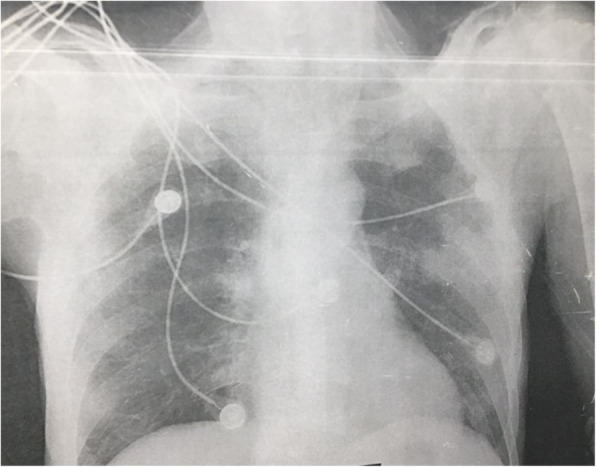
Chest X-ray (day 3) revealing multiple bilateral patchy lung consolidations and ground-glass opacities
